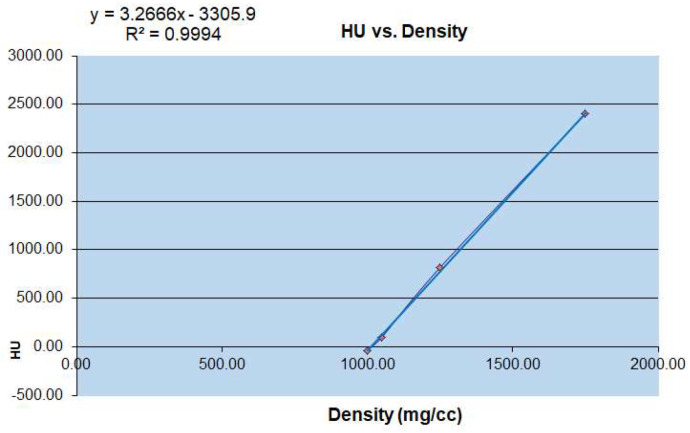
Conversion relationship between micro CT-HU value and bone mineral density (mg/cc).
